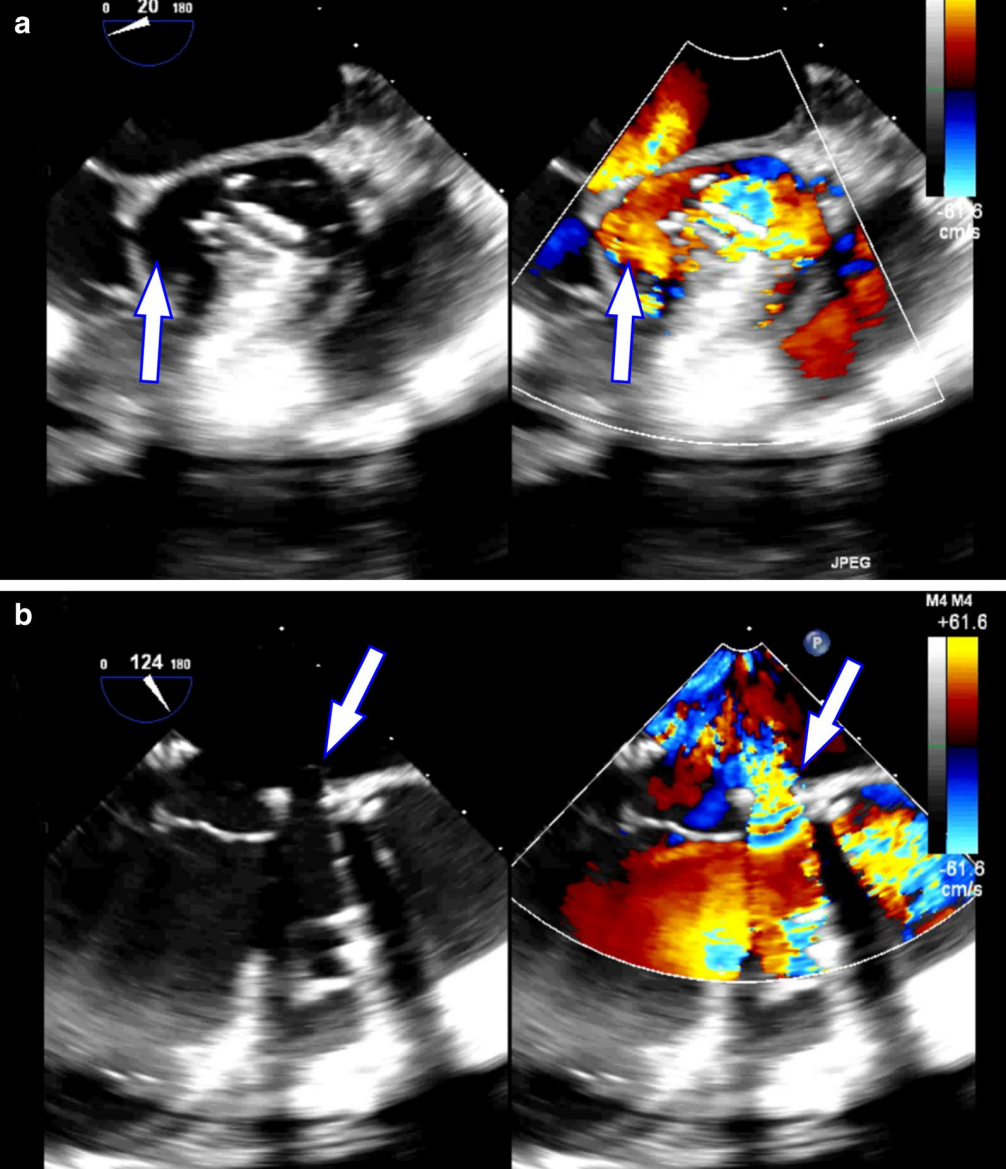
Echocardiography revealed prosthetic valve detachment, severe perivalvular leakage (a) and aorto–mitral curtain separation (b)
